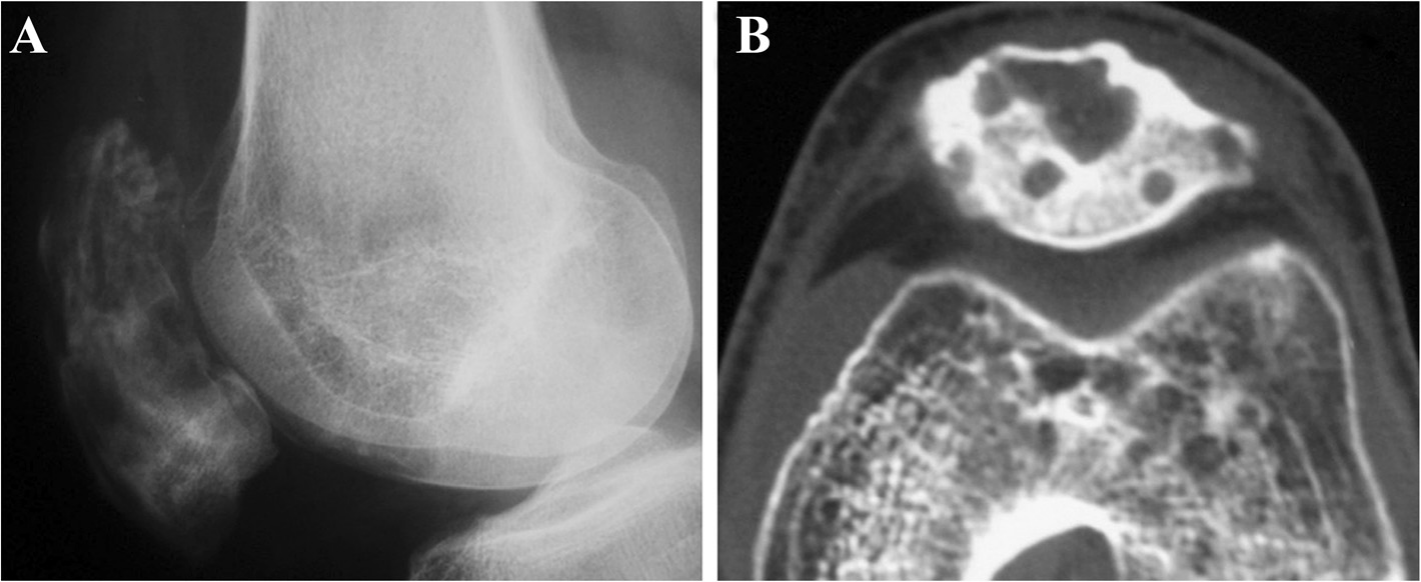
Hemangioendothelioma of the patella. Septation and thinning cortex in the osteolytic lesion are observed on (A) plain radiographs and (B) CT images.
